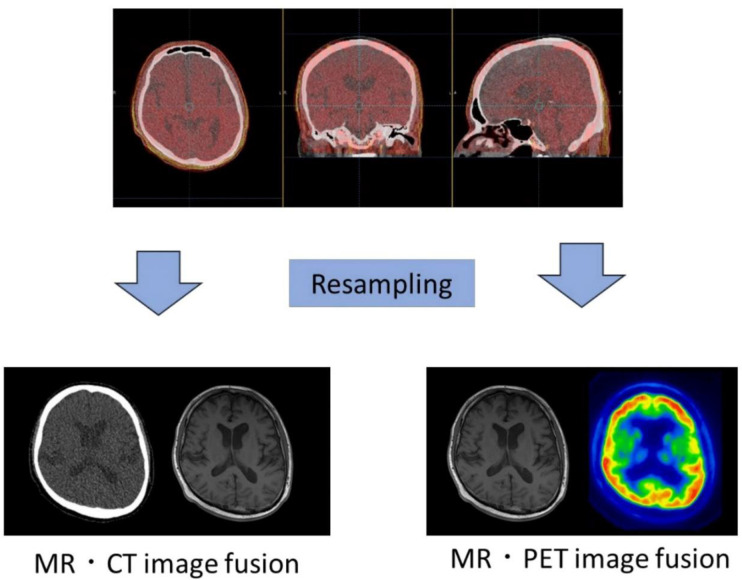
Image conversion and fusion. MRI, CT, and PET images were converted such that the matrix size and pixel size of the images were fused with each other. These images were reconstructed such that the anatomical positions coincided with each other.
